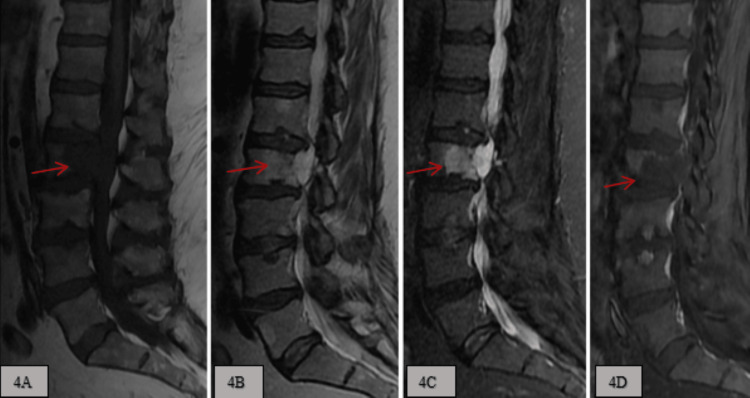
A: T1 weighted imaging, B: T2 weighted imaging, C: Short tau inversion recovery (STIR), D: T1 post-contrast imaging. These MRI sagittal image sequences demonstrate a 3.7 cm x 1.9 cm x 3.1 cm heterogenous expansile lesion at L2 vertebral body and pedicle, which is T1 hypointense, T2/STIR hyperintense, and subtle post-contrast enhancement. MRI: magnetic resonance imaging
